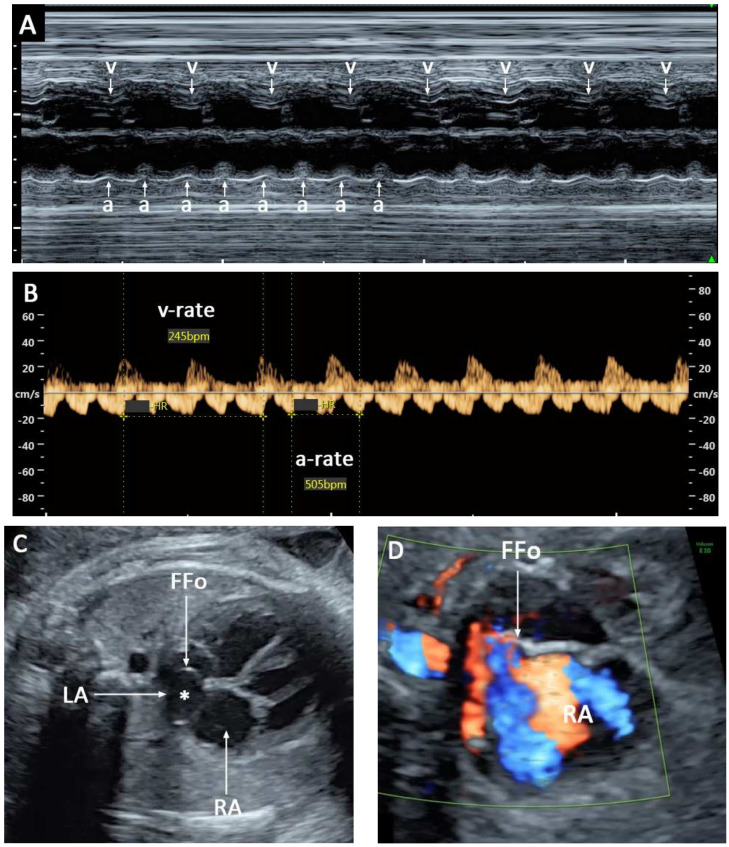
(A) M-mode shows a ventricular rate of (v) 245 bpm and an atrial rate of (a) 490 bpm; (B) Doppler waveforms of the renal vein (a-rate: 245 bpm) and renal artery (v-rate: 505 bpm); (C): Atrial septal aneurysm (*): wide excursion of the foramen ovale flap; (D): Color flow mapping in the septal aneurysm shows turbulent reversed flow returning back to the right atrium (FFo: flap of foramen ovale; LA: left atrium; RA: right atrium).
